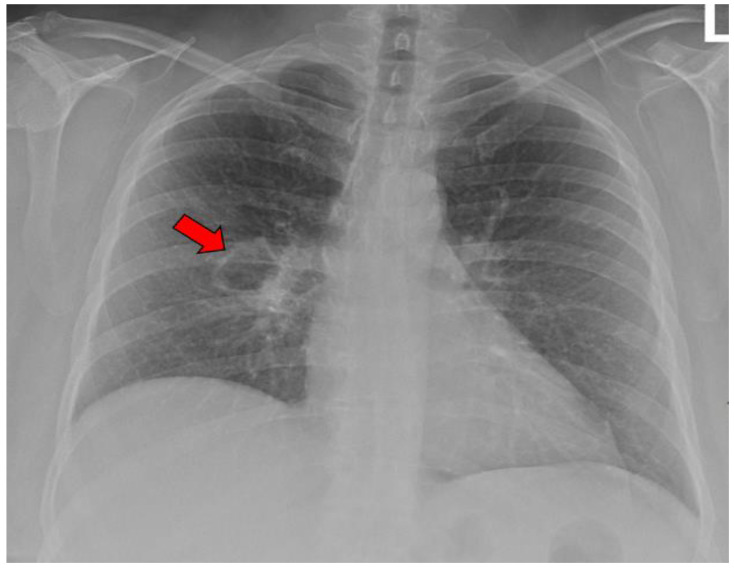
X-ray image of a single coccidioidal pulmonary cavity. The red arrow indicates a cavity.
